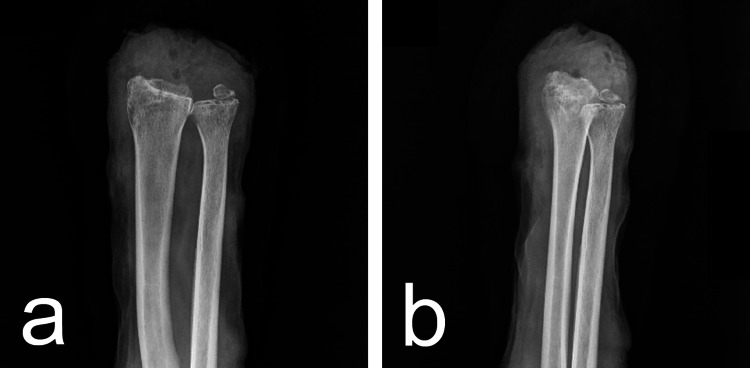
(a, b) Postoperative X-ray of the forearm.
